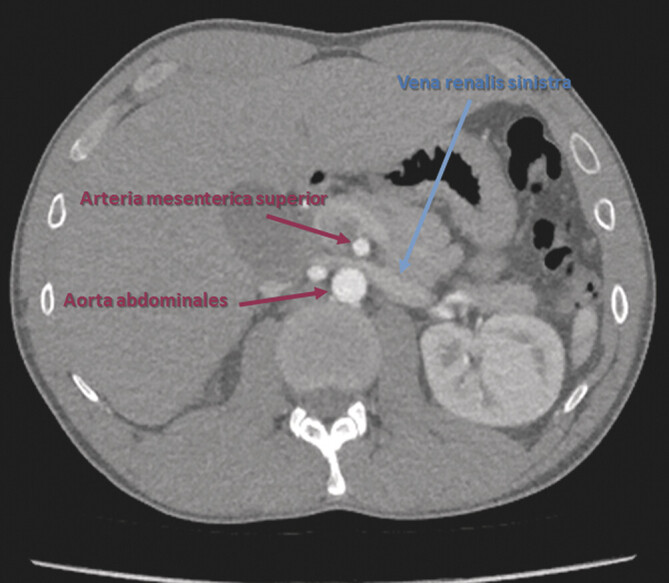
Angio-CT-Scan (axiale Rekonstruktion mit arterieller Kontrastmittelphase): „Schnabelzeichen“ – als schnabelartige Einengung der V. renalis sinistra zwischen A. mesenterica superior und Aorta abdominalis.
